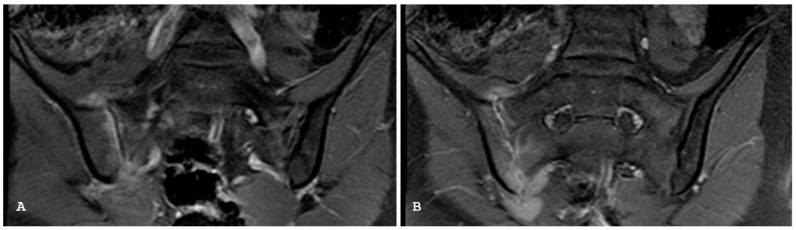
Oblique coronal T1 fat-saturated after gadolinium contrast magnetic resonance imaging sequences (A,B) demonstrate diffuse osteitis with associated synovitis, capsulitis, and periarticular inflammatory abscess in a 12-yr-old girl with clinic-laboratory signs of inflammation.
